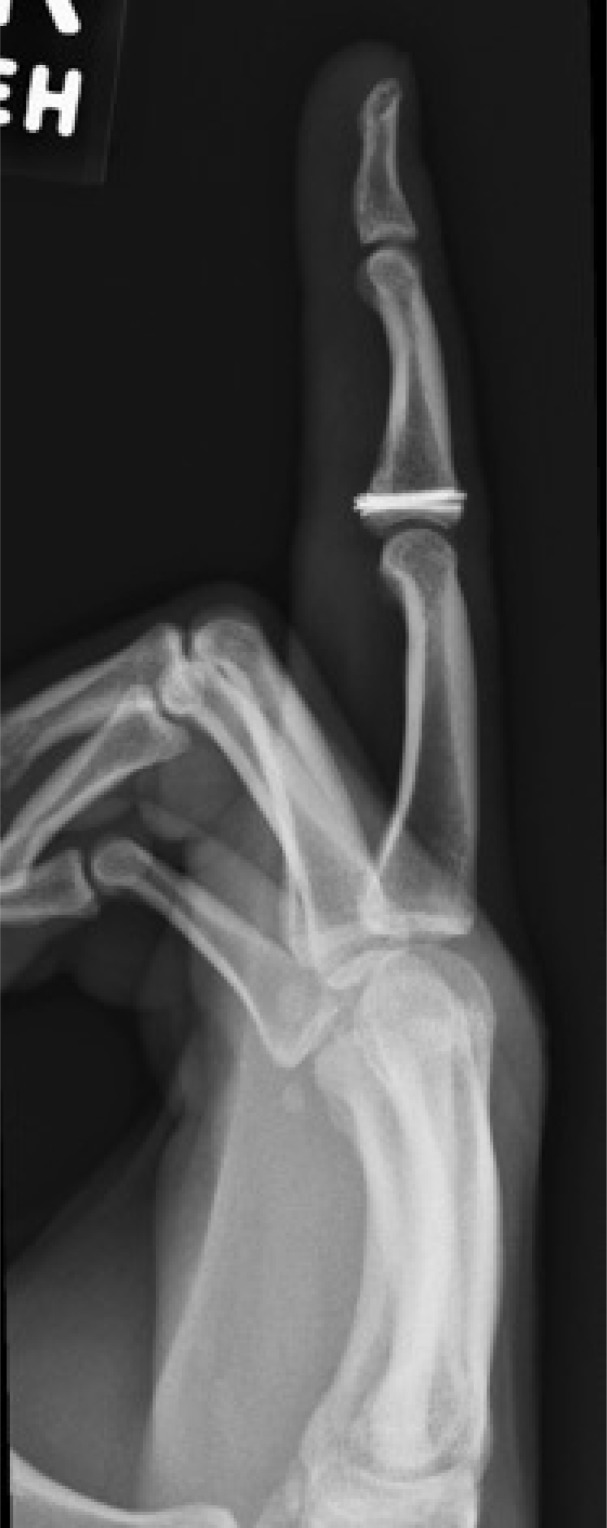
Patient 5 lateral radiograph at final follow-up.
